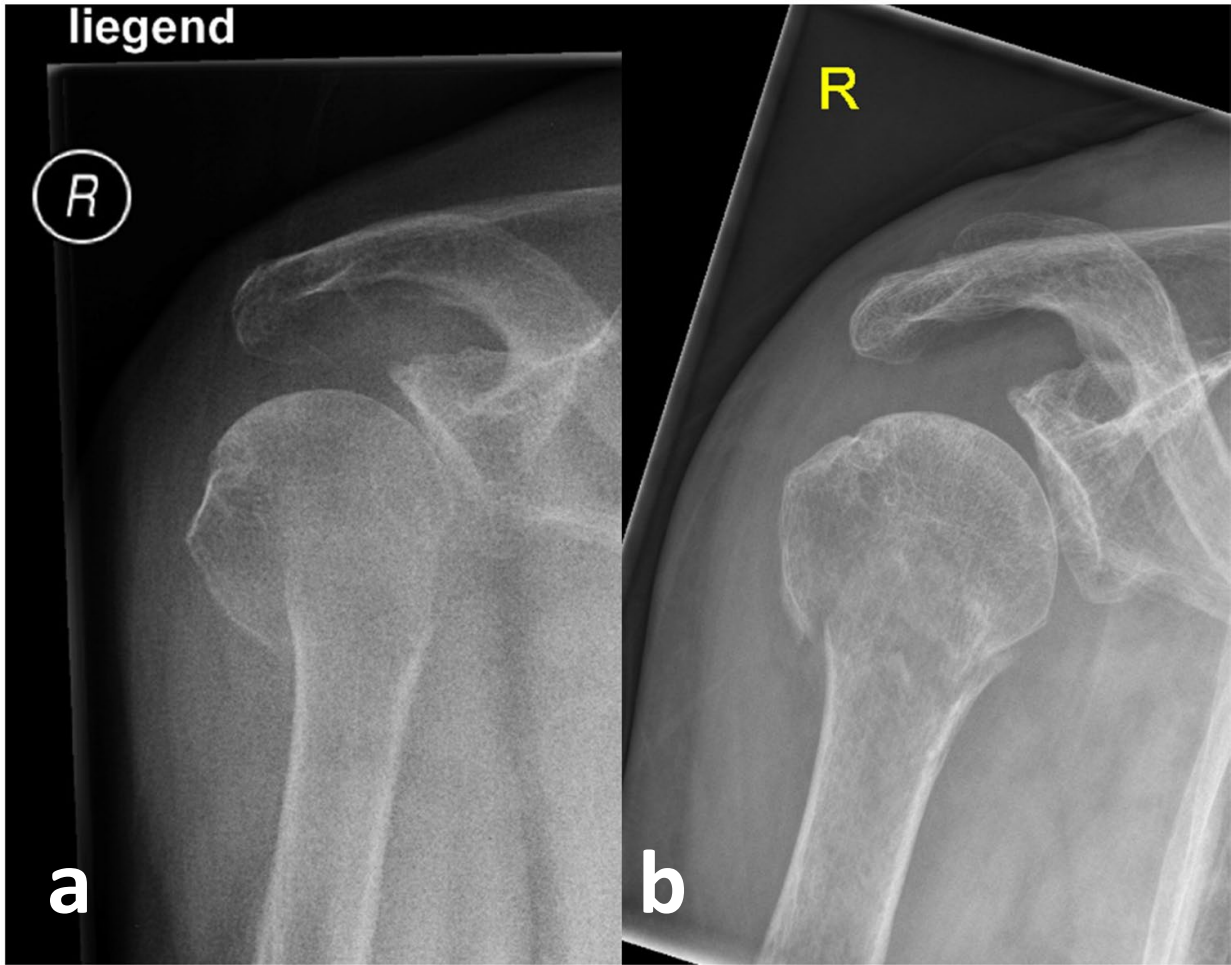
AP radiograph of an 85-year-old female patient on the day of injury in a lying supine position (a) and about 3 weeks later in a standing position with almost complete self-reducing of the presumed displacement that existed on the day of injury (b). Reprinted from *“Algorithmus zur konservativen Behandlung von proximalen Humerusfrakturen”* (https://link.springer.com/article/10.1007/s11678-022-00702-y, Copyright© 2022, Razaeian) under the terms of the Creative Commons CC BY 4.0 license (http://creativecommons.org/licenses/by/4.0/deed.de)
